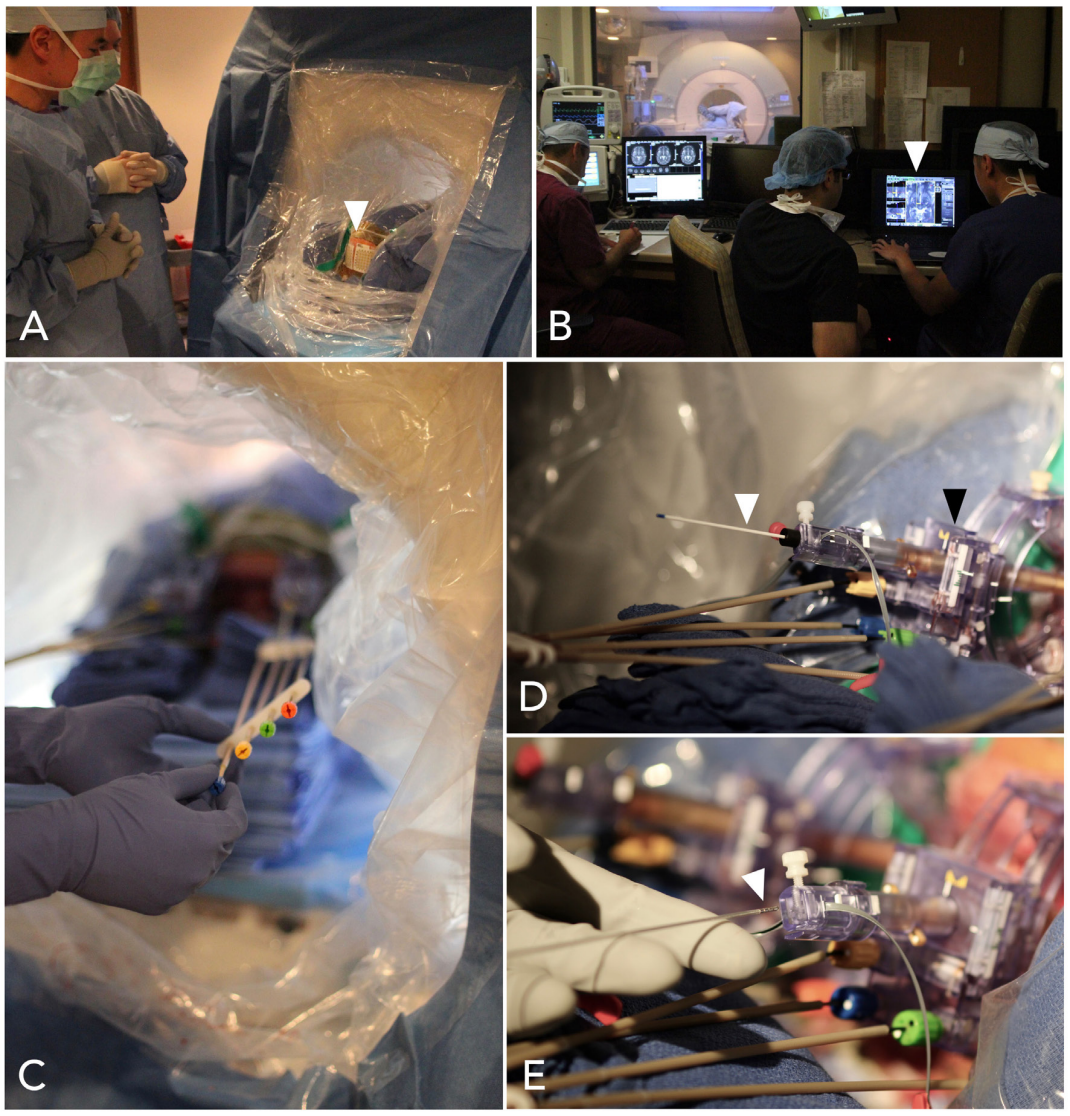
Steps in interventional-MRI-guided deep brain stimulation (DBS) lead implantation. (A) The patient is positioned supine and the head is sterilely draped within the MRI bore. MRI-visible marking grids (white arrowhead) are affixed to the scalp. (B) Volumetric scans are obtained and transferred to the ClearPoint planning station (white arrowhead), operated by the surgeon near the MRI console. (C) Instructions from the software are used to align the trajectories, by turning knobs affixed to a skull-mounted guide-frame. (D) When the guide-frame (black arrowhead) has been aligned such that the trajectory has a predicted radial error less than 0.5 mm, a ceramic stylet (white arrowhead) is placed to target depth, through a thin plastic sheath, and a scan is obtained to confirm accurate location. (E) A DBS lead (white arrowhead) is then placed through the sheath to target depth.
